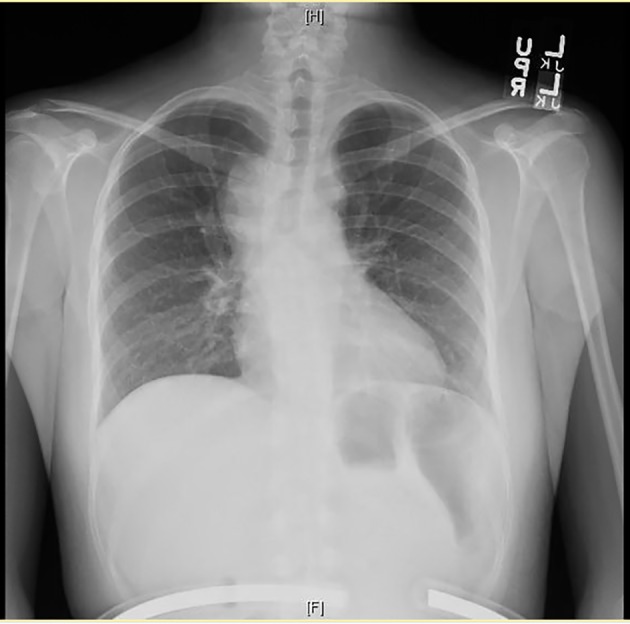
Chest X-ray showing right peri-hilar mass with tracheal compression.
